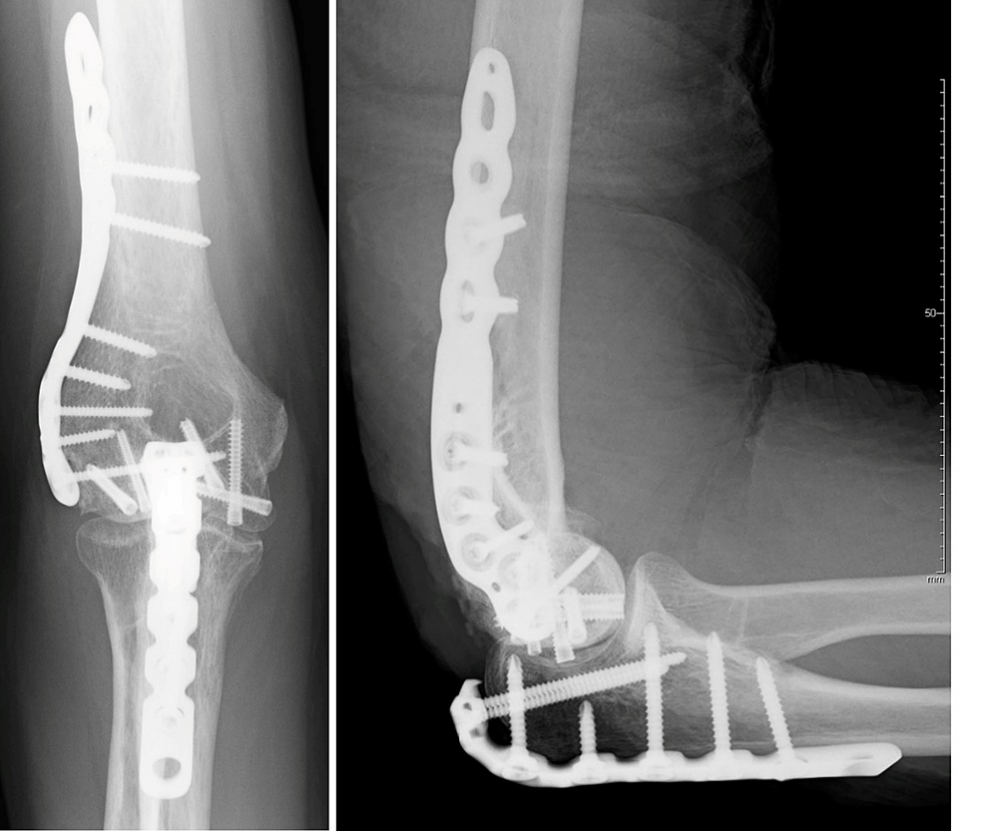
Postoperative radiograph showing obtaining anatomical reduction and stable fixation.
